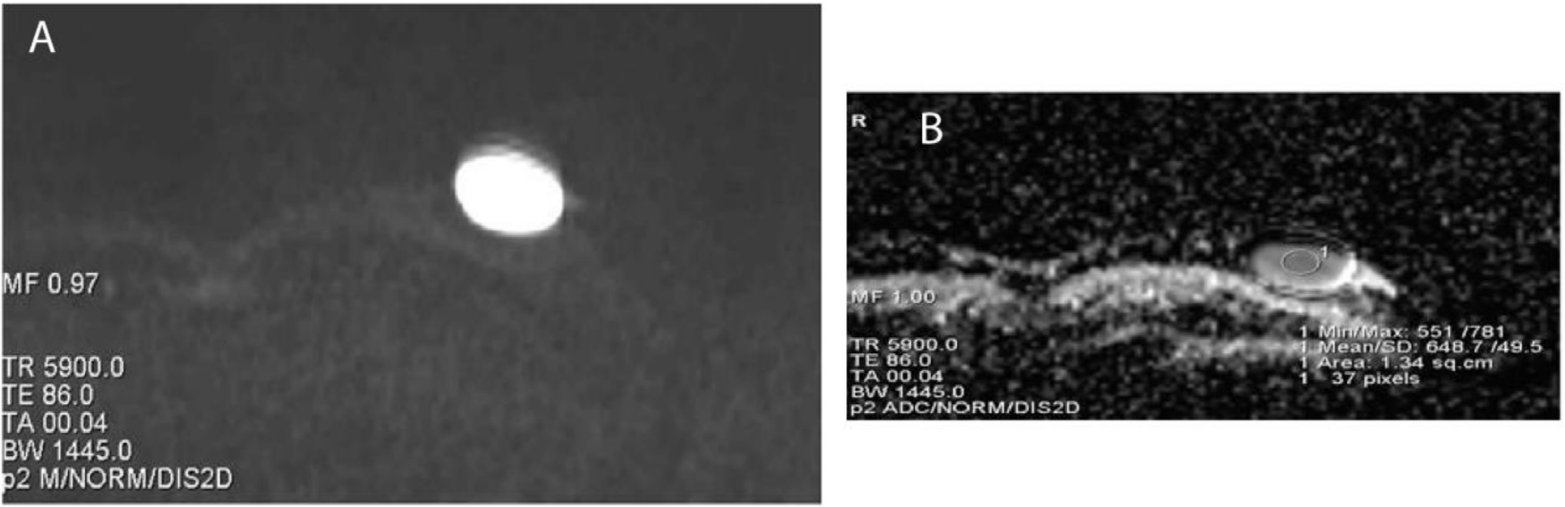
On diffusion weighted imaging (DWI), lesion showed markedly diffusion restriction with ADC value of 0.7 × 10^−3^ s/mm^2^ (A: DWI, B: ADC Map).
